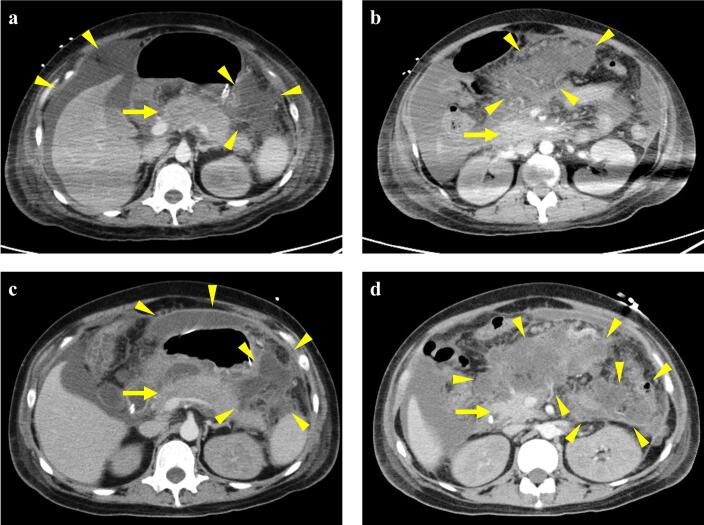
Contrast-enhanced computed tomography images. (a, b). On postpartum day 9, the pancreas appears enlarged (arrow), with surrounding fat stranding and peripancreatic and mesenteric fluid collection (arrowhead), which suggest acute pancreatitis. (c, d). On postpartum day 21, pancreatic enlargement (arrow) persisted without significant changes. Increased encapsulated peripancreatic fluid collections (arrowhead), with areas of internal heterogeneity suggestive of evolving necrosis, consistent with acute necrotic collections.
